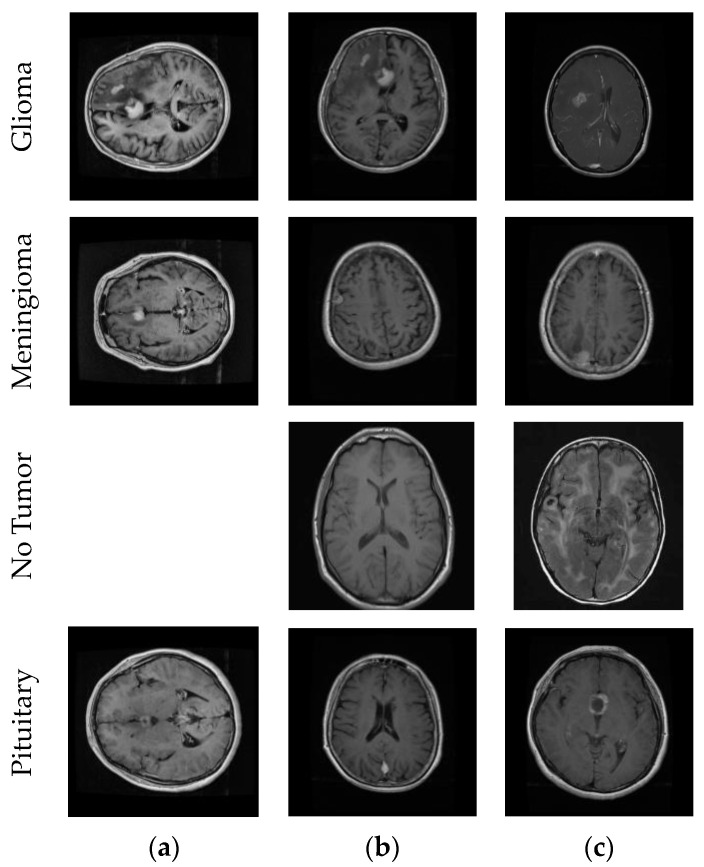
Sample MR images from the datasets. (a) Dataset 1; (b) Dataset 2; (c) Dataset 3.
